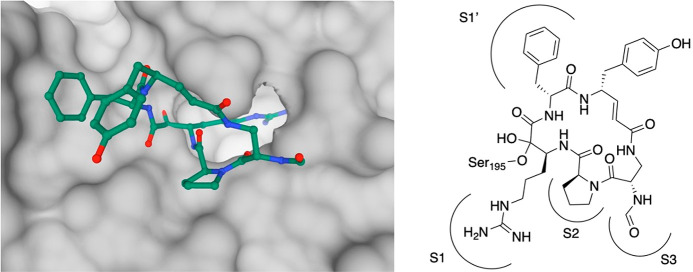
X-ray structure of trypsin in complex with cyclotheonamide
